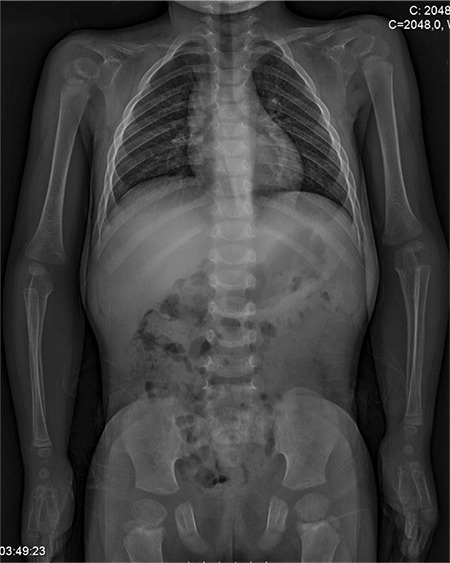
X-ray of her trunk shows bilateral hypoplastic clavicles, hypoplasia of iliac bones, wide symphysis pubis
